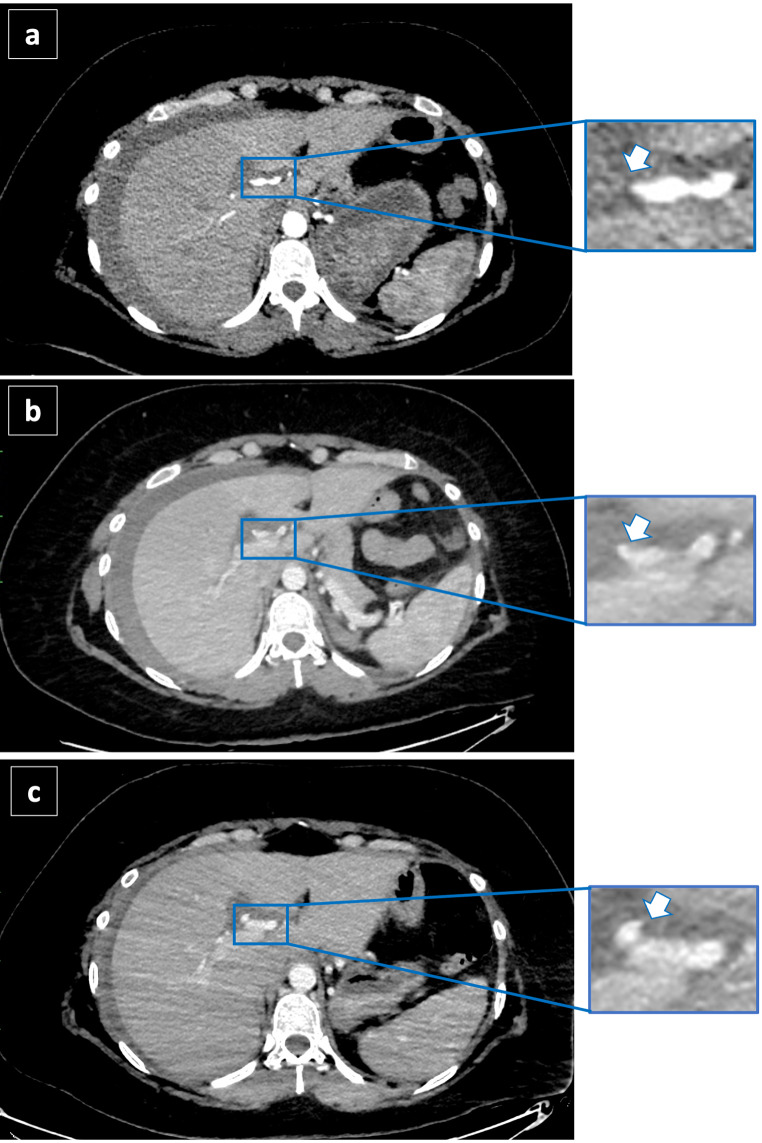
Contrast - enhanced CT after iodinated contrast agent injection during arterial phase on arrival at the Emergency Department (a) 20-hours after admission (b) and 5 days after admission at the Emergency Department (c). The contrast leakage (arrow) at the level of hepatic artery becomes progressively larger from 2 mm (a), up to 4 mm (b) and 7 mm (c) and becomes progressively more evident
